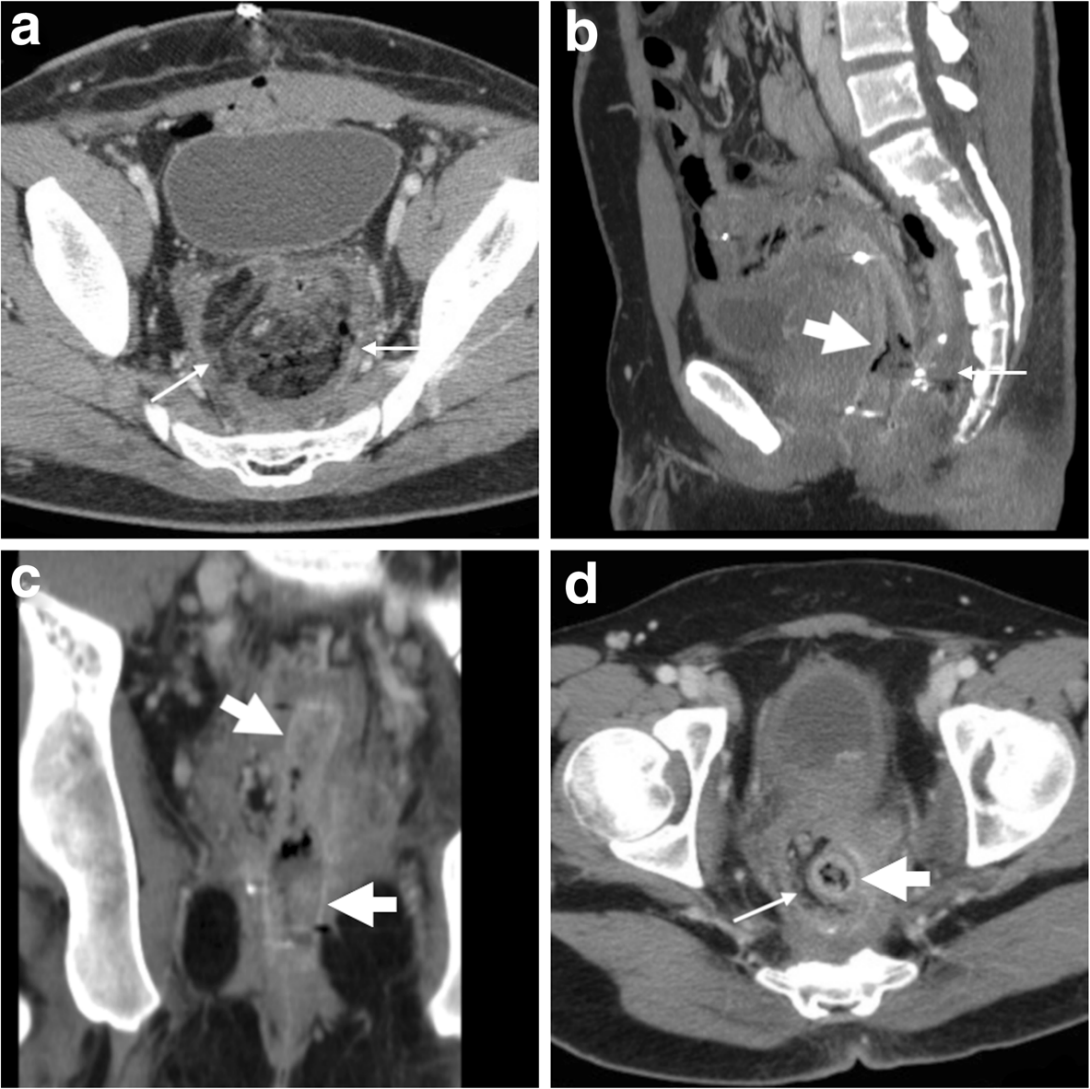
Use of bioabsorbable stent to manage a chronic anastomotic fistula following anterior rectal resection. After drainage of a perianastomotic abscess (not shown), CT (a) showed the persistence of perianastomotic inflammation (thin arrows) with gas bubbles (note the close similarity to Fig. 11b). Repeated CT (b–d) showed decreased perianastomotic inflammation (thin arrows) compared to a and confirmed correct placement of the bioabsorbable stent (thick arrows) which appears as a tubular structure with soft-tissue attenuation, best visible using MIP reconstructions (b).
